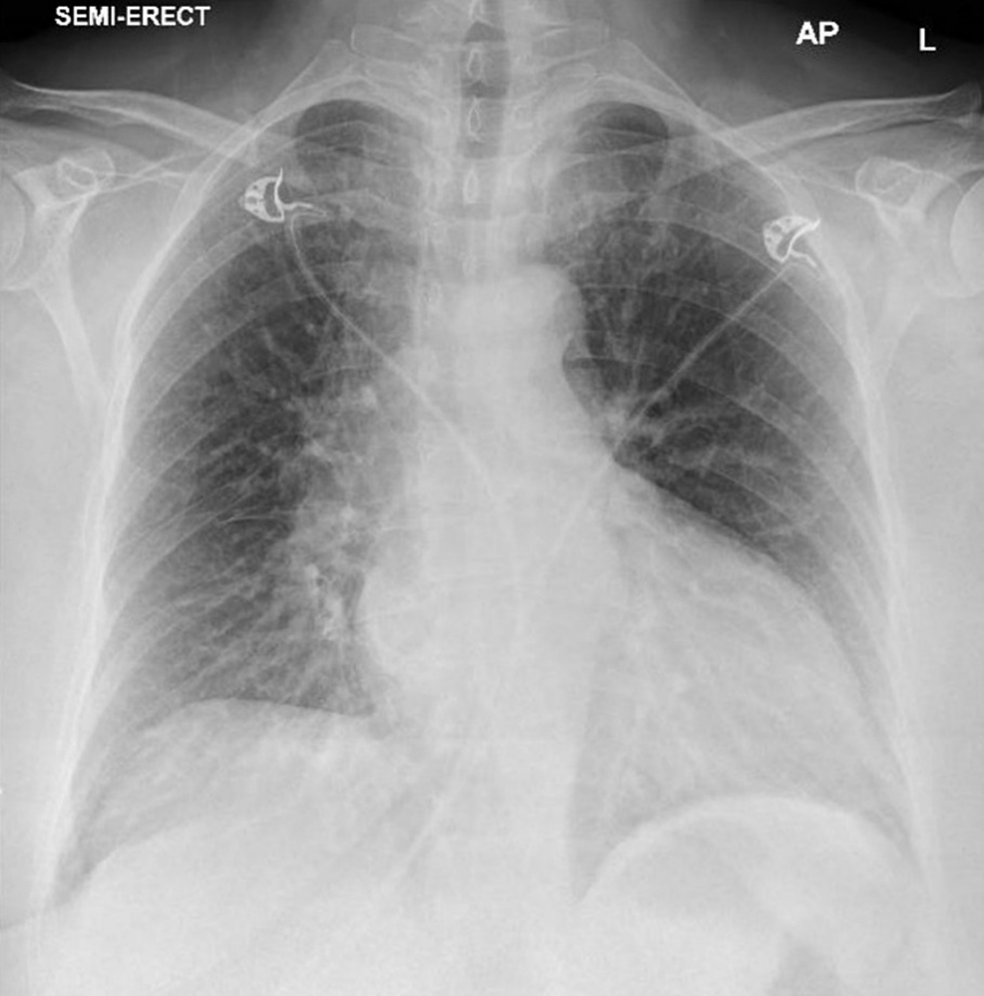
Semi-erect AP chest x-ray shows prominent pulmonary vasculature and congested lung fields.
